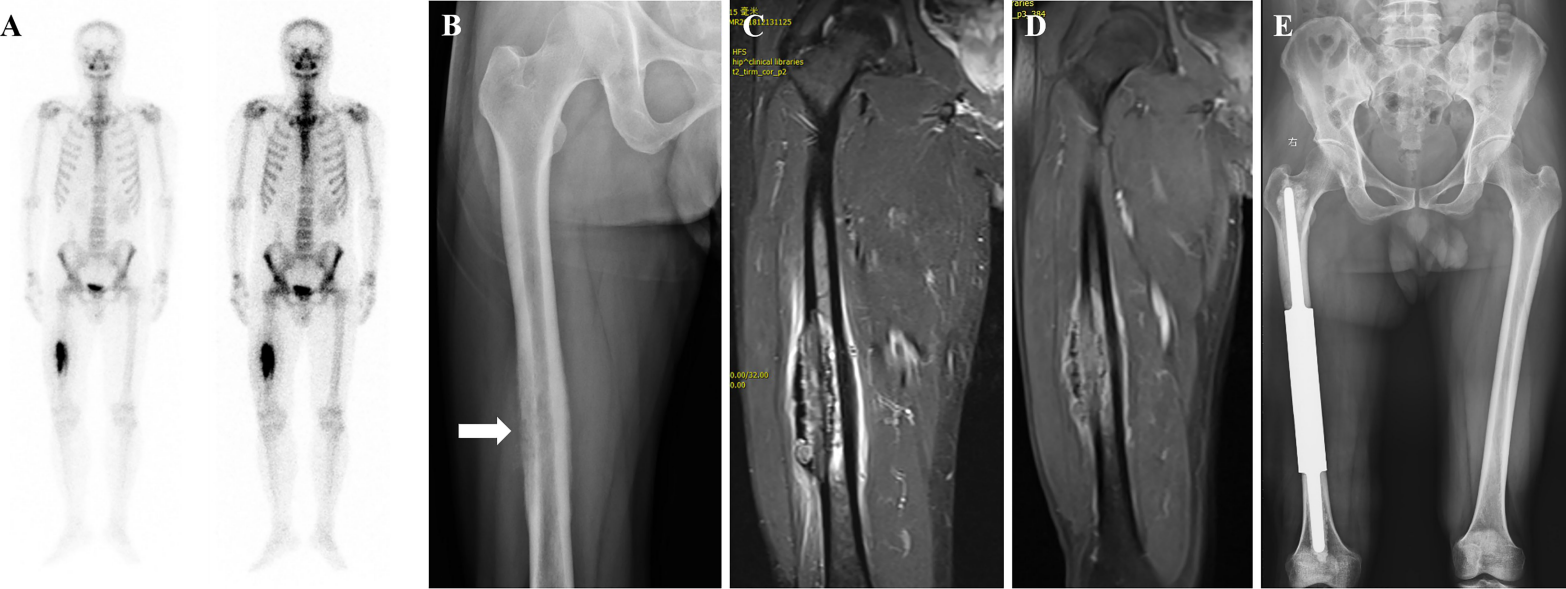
A patient with isolated metastasis of the right femoral shaft. (A) Emission Computed Tomography showing an isolated metastatic lesion in the right femoral shaft with active metabolism. (B) Radiograph showing osteolytic destruction of the right femoral shaft. (C, D) Magnetic resonance image showing decreased T1-weighted image signal and increased T2-weighted image signal, consistent with the diagnosis of osteolytic bone metastases. (E) Post-operative radiograph of customized modular prosthesis implantation.
